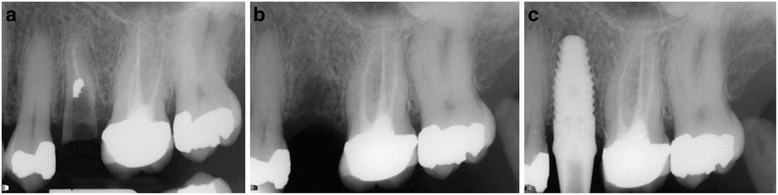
Radiographic imagines. a preoperative view, (b) 60 days post grafting; (c) final implant placement
